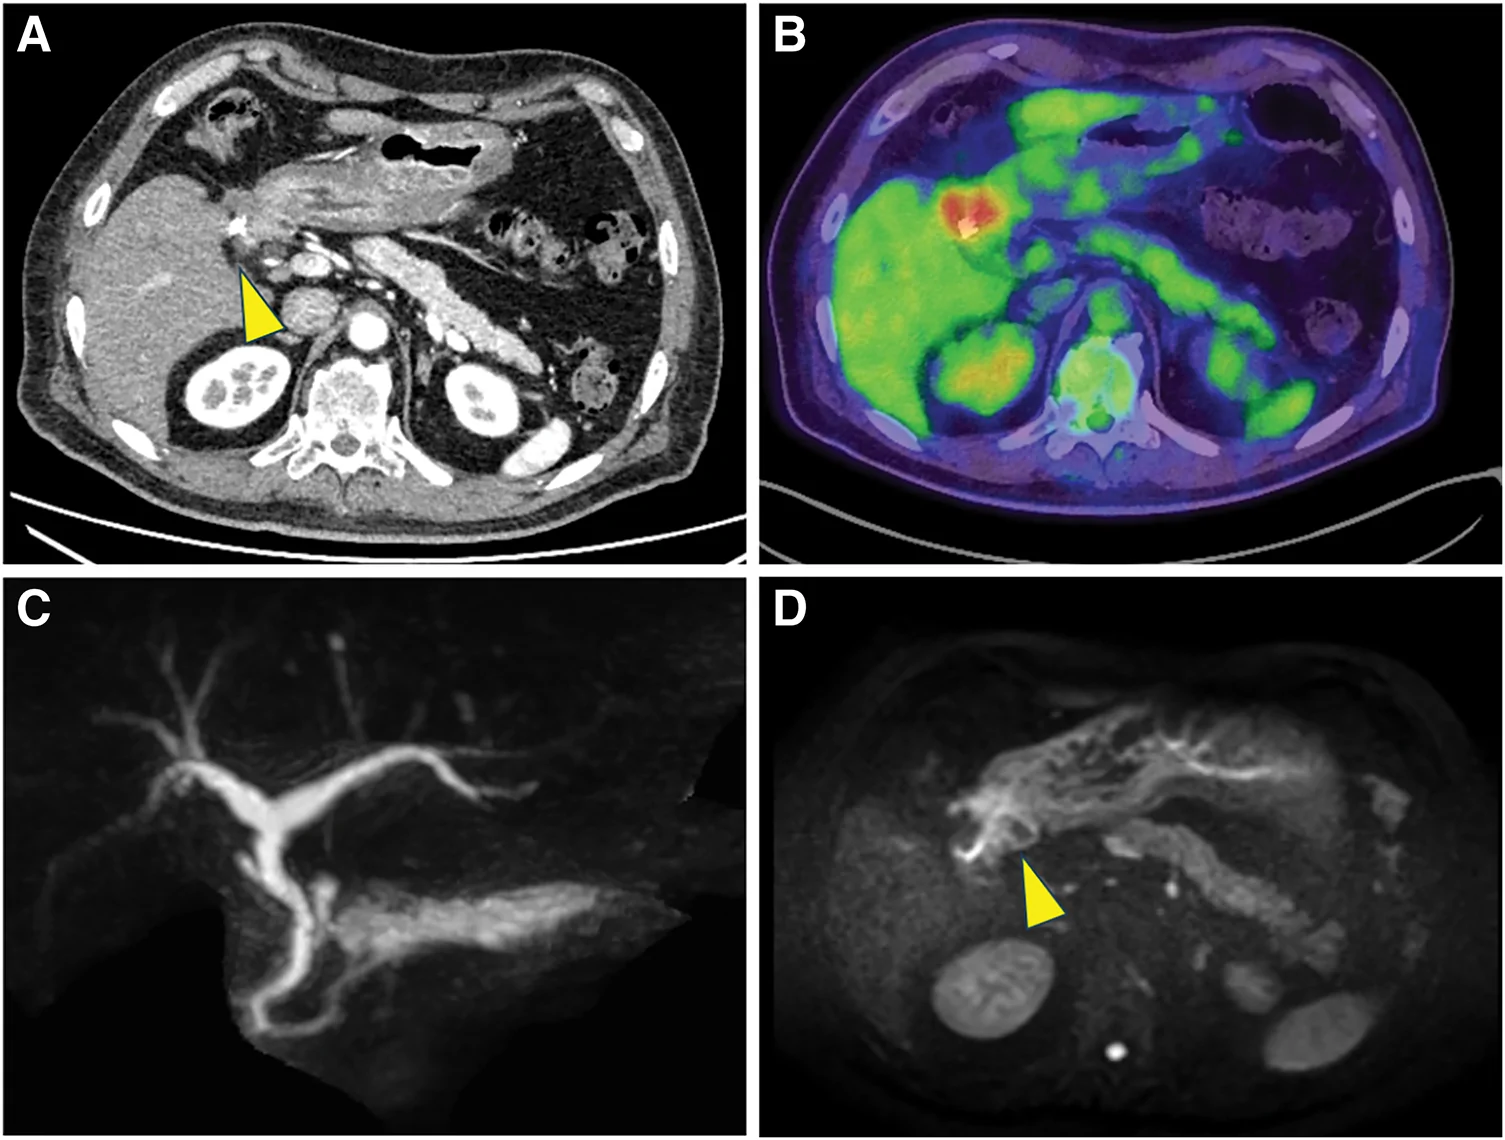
Contrast-enhanced abdominal CT, ^18^F-FDG PET images, and MRCP obtained before SSPPD. (A) The arrowhead indicates the area where the tumor is invading the liver. (B) ^18^F-FDG PET shows abnormal FDG uptake corresponding to the lesion (SUVmax: 6.757). (C) There is no dilation of the common bile duct or the main pancreatic duct. (D) The arrowhead indicates diffusion restriction in the duodenal wall. FDG, fluorodeoxyglucose; MRCP, magnetic resonance cholangiopancreatography; SSPPD, subtotal stomach-preserving pancreaticoduodenectomy
